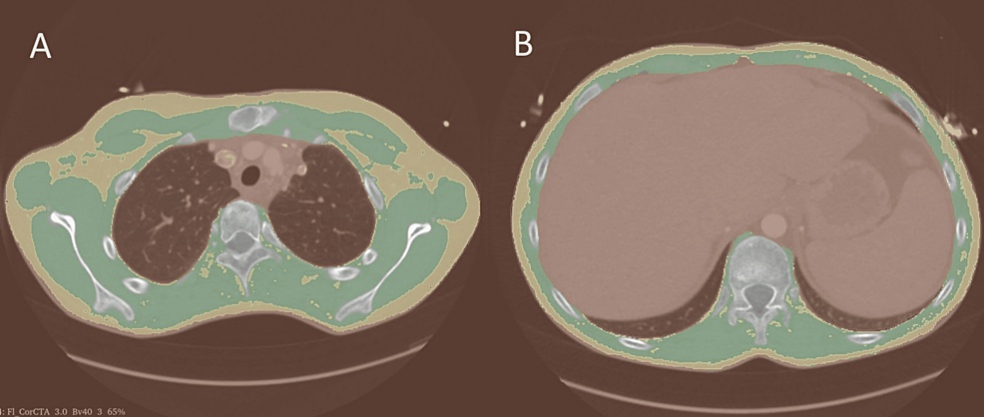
Axial view at the level of the 4th thoracic vertebra (A) and 12th thoracic vertebra (B), segmentation for calculation of SMA (green) and subcutaneous fat mass (ochre), CT SMA: skeletal muscle area, CT: computed tomography
